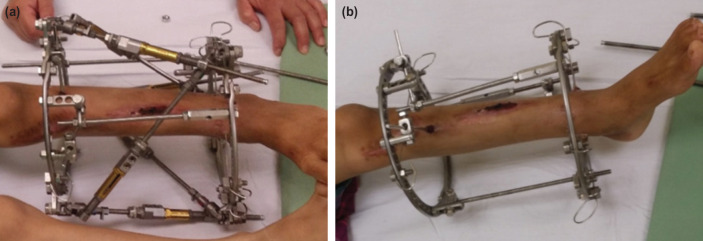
(a) Triple hinge pillar attached on the SUV frame prior to removal of struts. (b) All struts have been removed and replaced with three triple hinge pillars.
